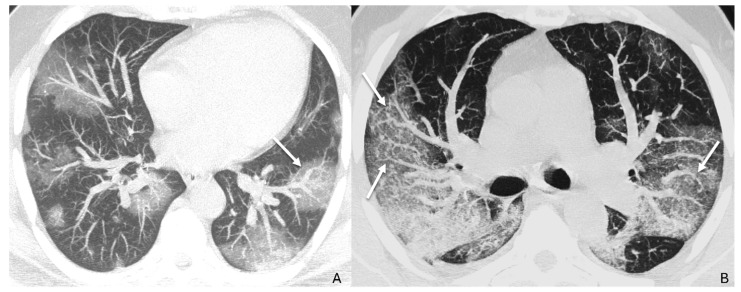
CT images, optimized lung window, MIP reconstruction. (A) Dilated segmental and subsegmental pulmonary vessels in the context of an area of ground glass (arrow); please make a comparison with comparable regions of non-diseased lung parenchyma. (B) Dilated and tortuous peripheral pulmonary vessels with a branching aspect resembling a budding tree and consistent with the vascular tree-in-bud pattern (arrows).
